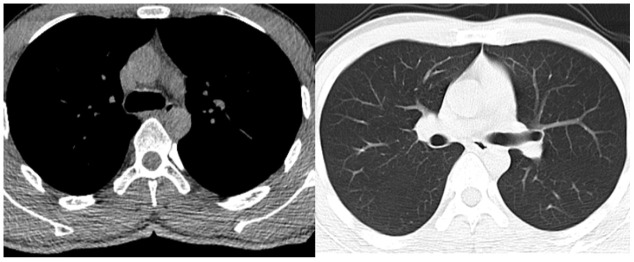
Preoperative chest CT examination of the patient.
